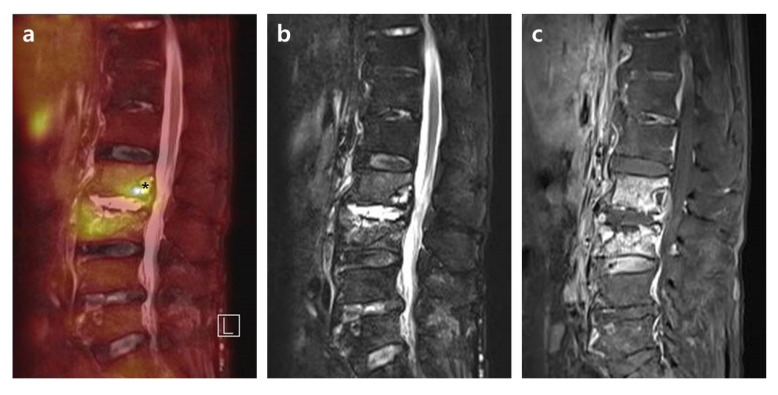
Simultaneous FDG-PET/MRI with the location of SUV_max_ on the vertebral body after successful antimicrobial therapy. A 77-year-old female patient shows PVO of L2–3 on simultaneous FDG-PET/MRI with SUV_max_ 5.99 on the vertebral body (asterisk) after 31 days of vancomycin (CRP 0.49 mg/dL). In the distribution pattern of the PVO lesion, FDG uptake on FDG-PET (a) shows the highest FDG uptake on the vertebral body of L2 and diffuse FDG uptake on L3. High signal intensity on T2FS (b) also shows similar distribution pattern of FDG-PET. However, there are significantly increased enhancements and high signal intensity on the overall PVO lesion on T1C (c). FDG-PET/MRI, ^18^F-fluorodeoxyglucose positron emission tomography/magnetic resonance imaging; SUV_max_, maximum standardized ^18^F-fluorodeoxyglucose uptake value; PVO, pyogenic vertebral osteomyelitis; CRP, C-reactive protein; T2FS, T2-weighted fat saturation magnetic resonance imaging; TIC, T1-weighted contrast magnetic resonance imaging.
